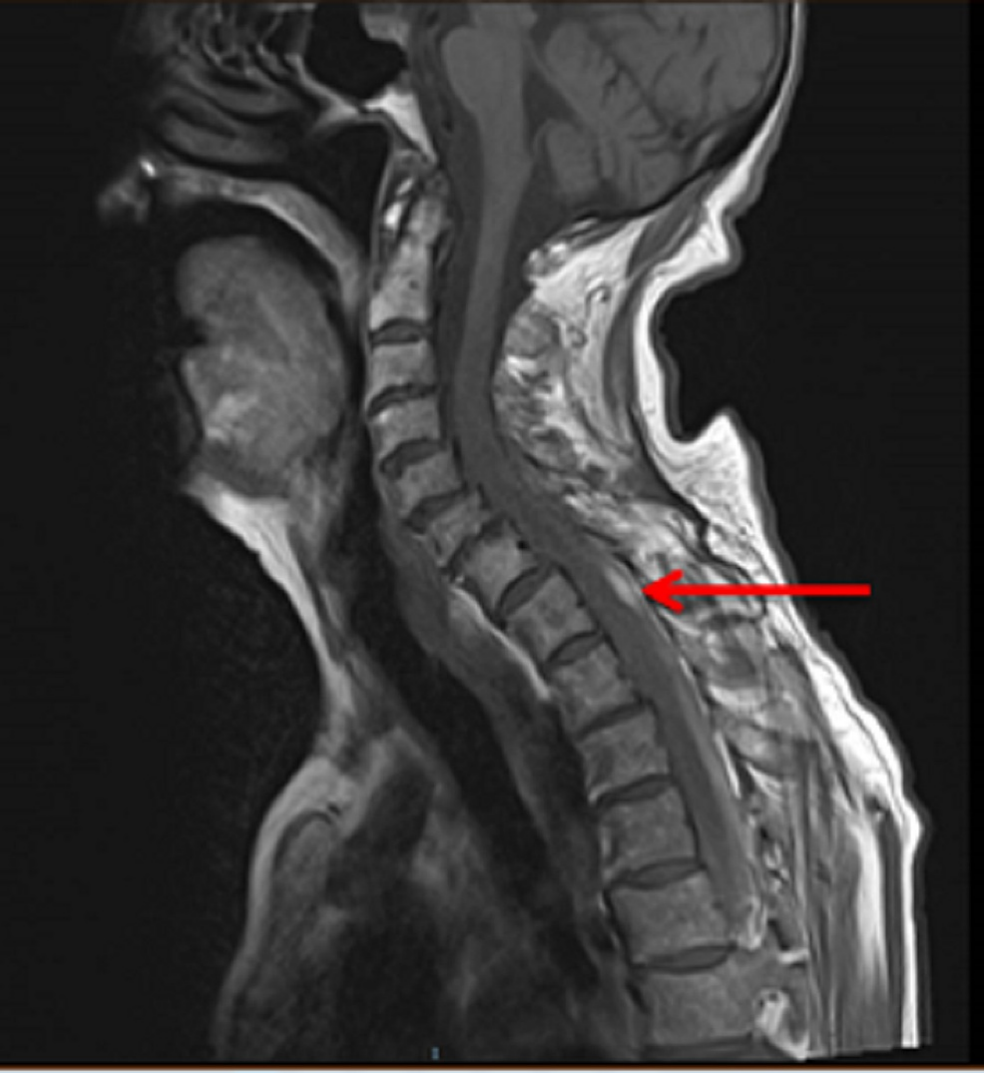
Sagittal T1-weighted sequence cervical and upper thoracic spine. Normal CSF is of low signal intensity and this is seen within the spinal canal at the cervical spine level. The spinal subarachnoid haemorrhage is demonstrated as abnormal high signal intensity posterior to the cord at the level of the T1 vertebral body (red arrow), this extends caudally all the way down the spinal canal and is seen within the distal thoracic and lumbar spine. CSF: cerebrospinal fluid.
